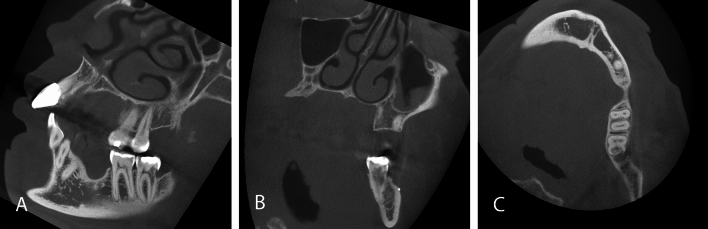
CBCT scan of a 41-year-old female patient with a large FOV (10 × 10 cm) with multi-unit gaps. A cervical root resorption was discovered as an incidental finding on the second lower left molar. Furthermore, in the coronal plane (B) a small radiopaque concrement is visible on the buccal bone as well as circumferential thickening of the maxillary sinus mucosa in the left maxillary sinus. Sagittal (A), coronal (B) and axial (C) images
